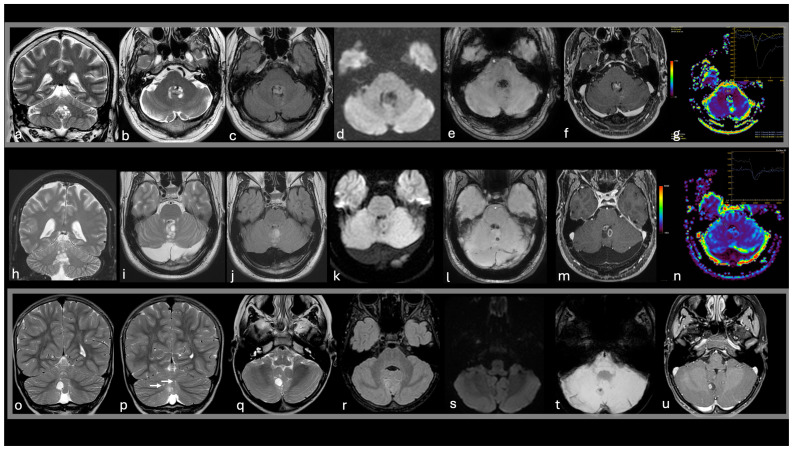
Differential diagnoses of MVNT in the infratentorial compartment. T2w coronal images (a,h,o,p), T2w axial images (b,i,q), FLAIR axial images (c,j,r), DWI (d,k,s), SWI (e,l,t), post-contrast T1w (f,m,u), DSC-CBV maps/time curves (g,n).
